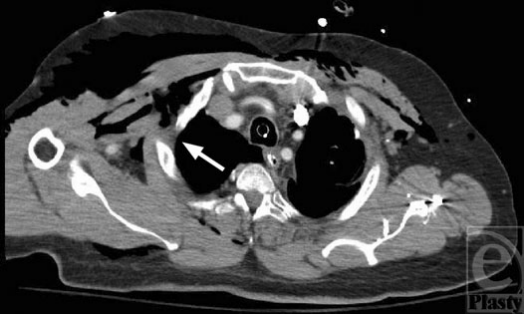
Computed tomography imaging at the second intercostal space shortly after chest tube insertion demonstrating subcutaneous emphysema due to persistent air leak originating from sites of repeated needle thoracostomy attempts (white arrow). The air leak was exacerbated by high peak airway pressures required to maintain oxygenation and ventilation.
